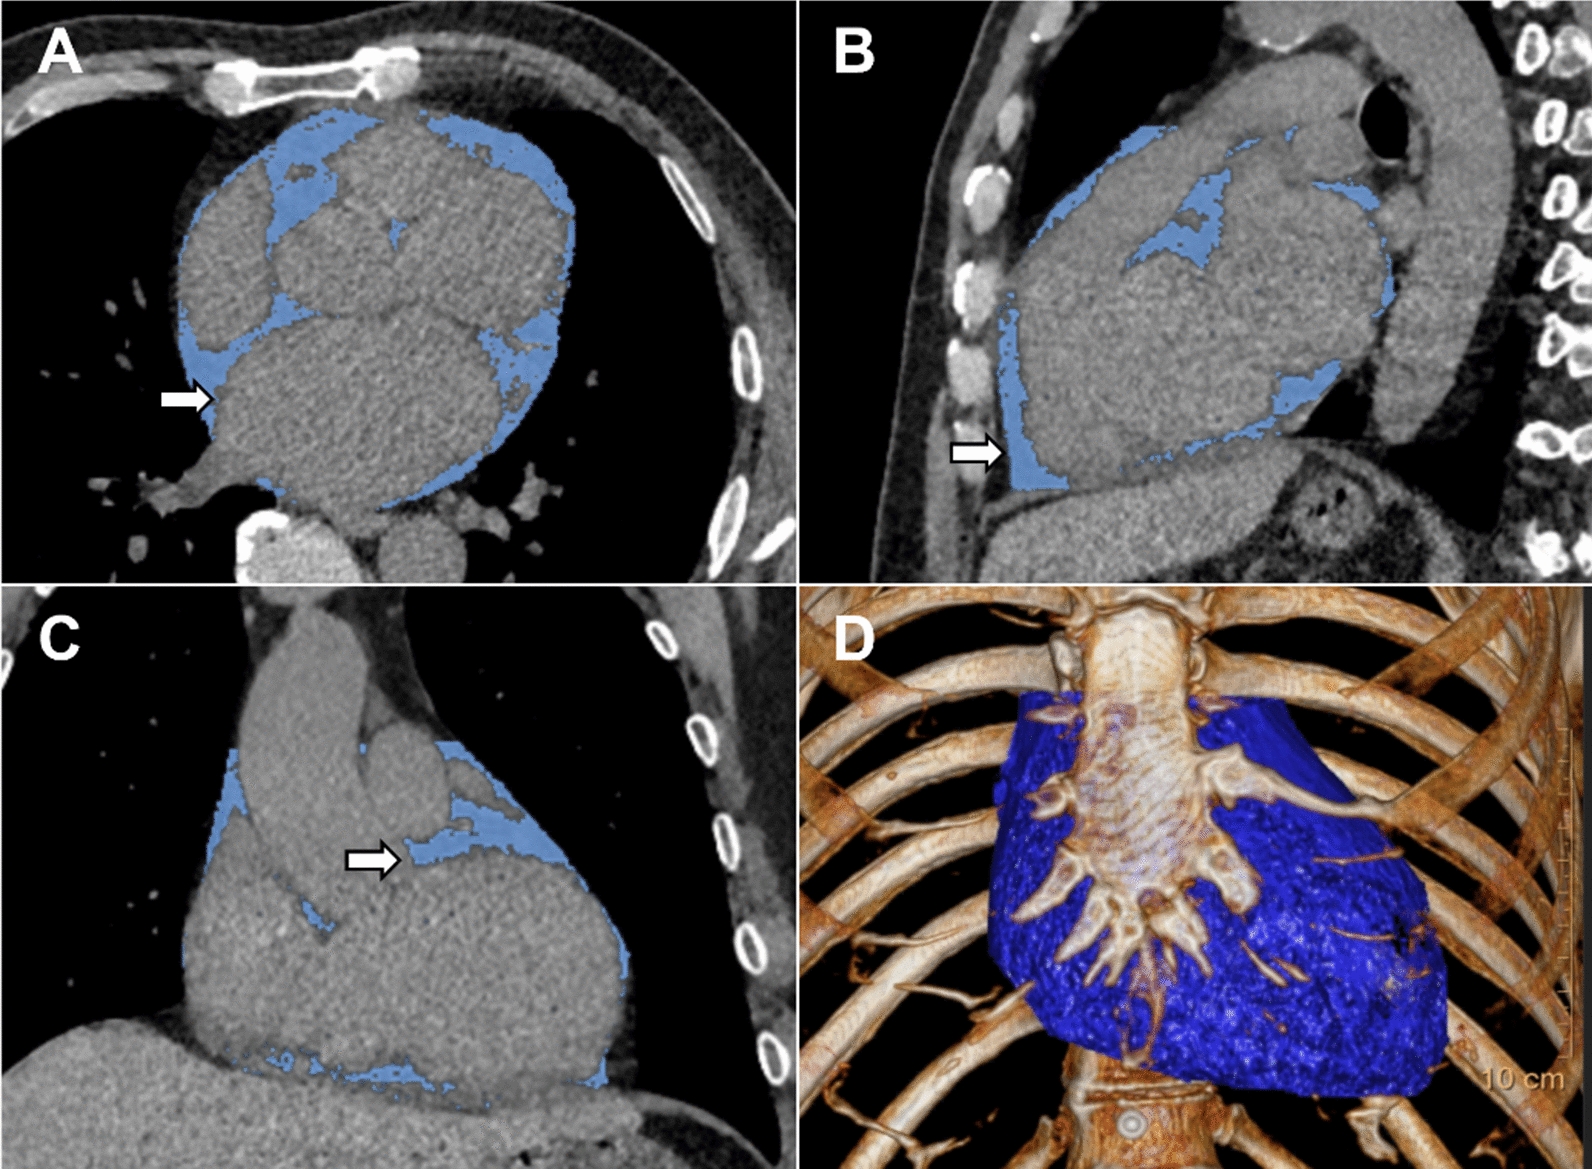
Epicardial adipose tissue on computed tomography. Axial (A), sagittal (B), and coronal (C) images of epicardial adipose tissue quantification. Adipose tissue is highlighted in blue color and pointed out with white arrows. D A 3-D reconstruction of epicardial adipose tissue
